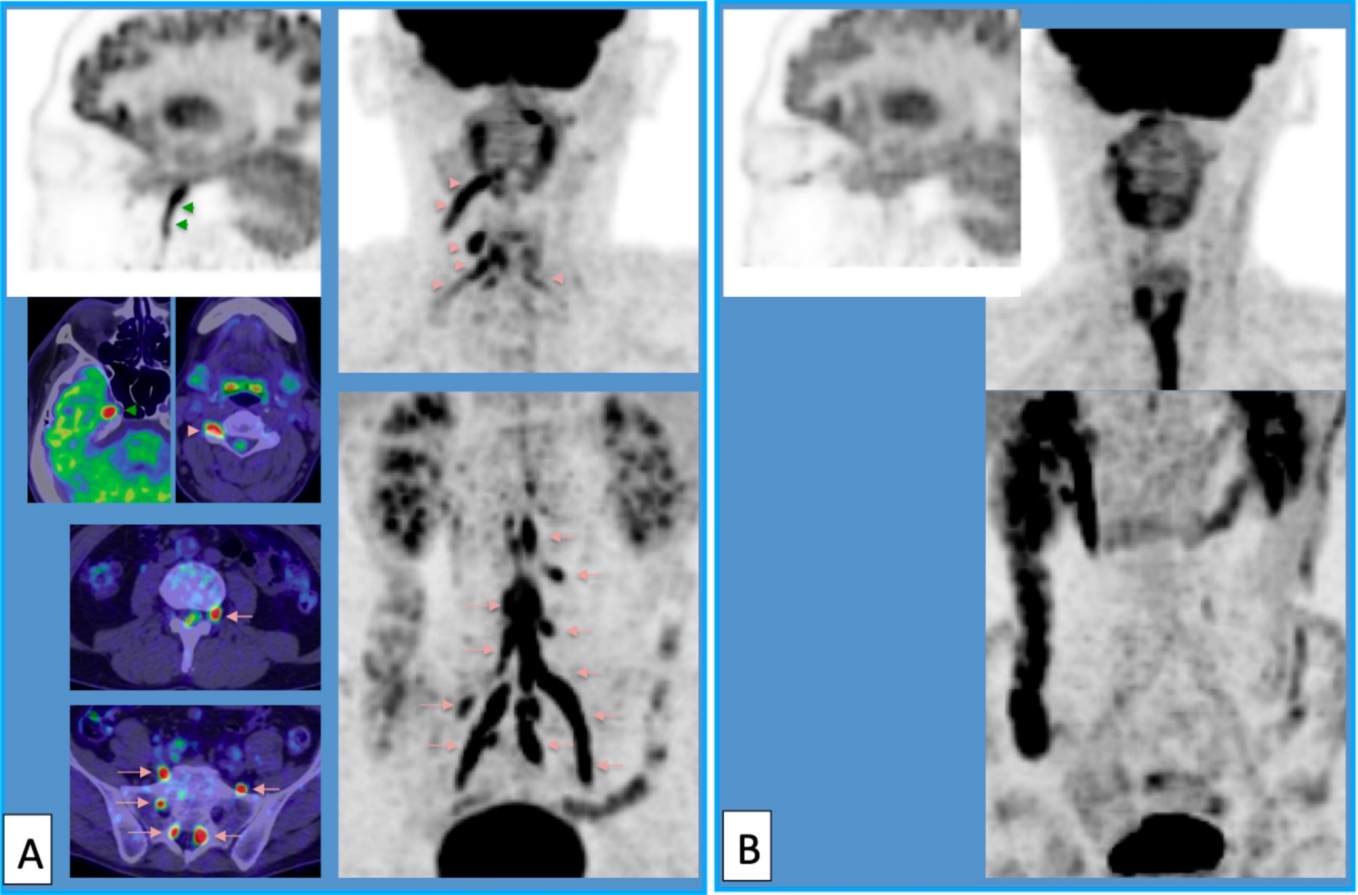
High magnification of fluorodeoxyglucose positron emission tomography (FDG-PET) after chemotherapy and after craniospinal irradiation High magnification of FDG-PET after chemotherapy with high-dose methotrexate (MTX) (A) and after irradiation (B). Accumulation of FDG developed in the right trigeminal nerve (green arrowheads) and cervical (red arrowheads) and lumbosacral (red arrows) roots and plexus (A). These high-uptake areas disappeared after irradiation (B).
